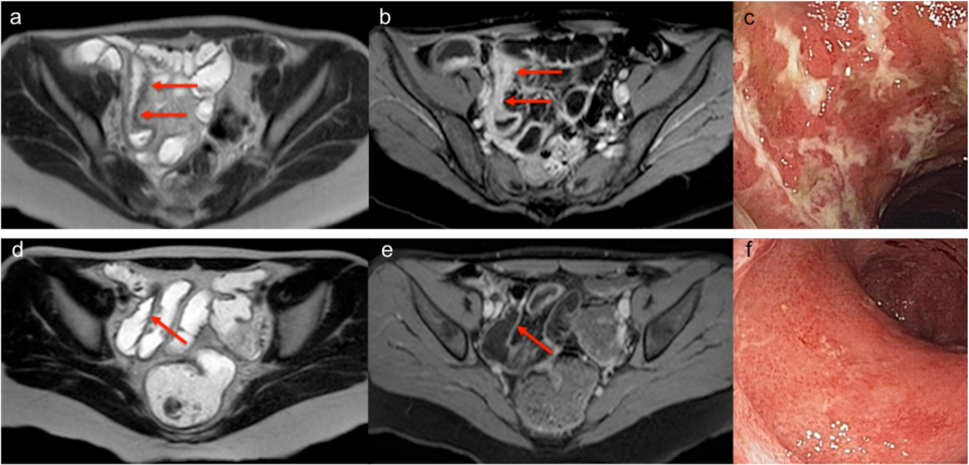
Patient with CD of the terminal ileum in treatment with IFX. At baseline, MRI (a and b) detected moderate inflammatory lesions of the terminal ileum, with wall thickening accompanied by oedema, irregularity of the mucosal surface and hyperenhancement after intravenous contrast administration (Overall MaRIA score = 49). Baseline endoscopy of the same segment confirmed the presence of serpiginous, longitudinal ulcerations (c; SES-CD = 20). At week 26, the terminal ileum achieved healing at both MRI (d and e; Overall MaRIA score = 19,2; Δ MaRIA score = 29,8) and endoscopy (f; SES-CD = 0)
